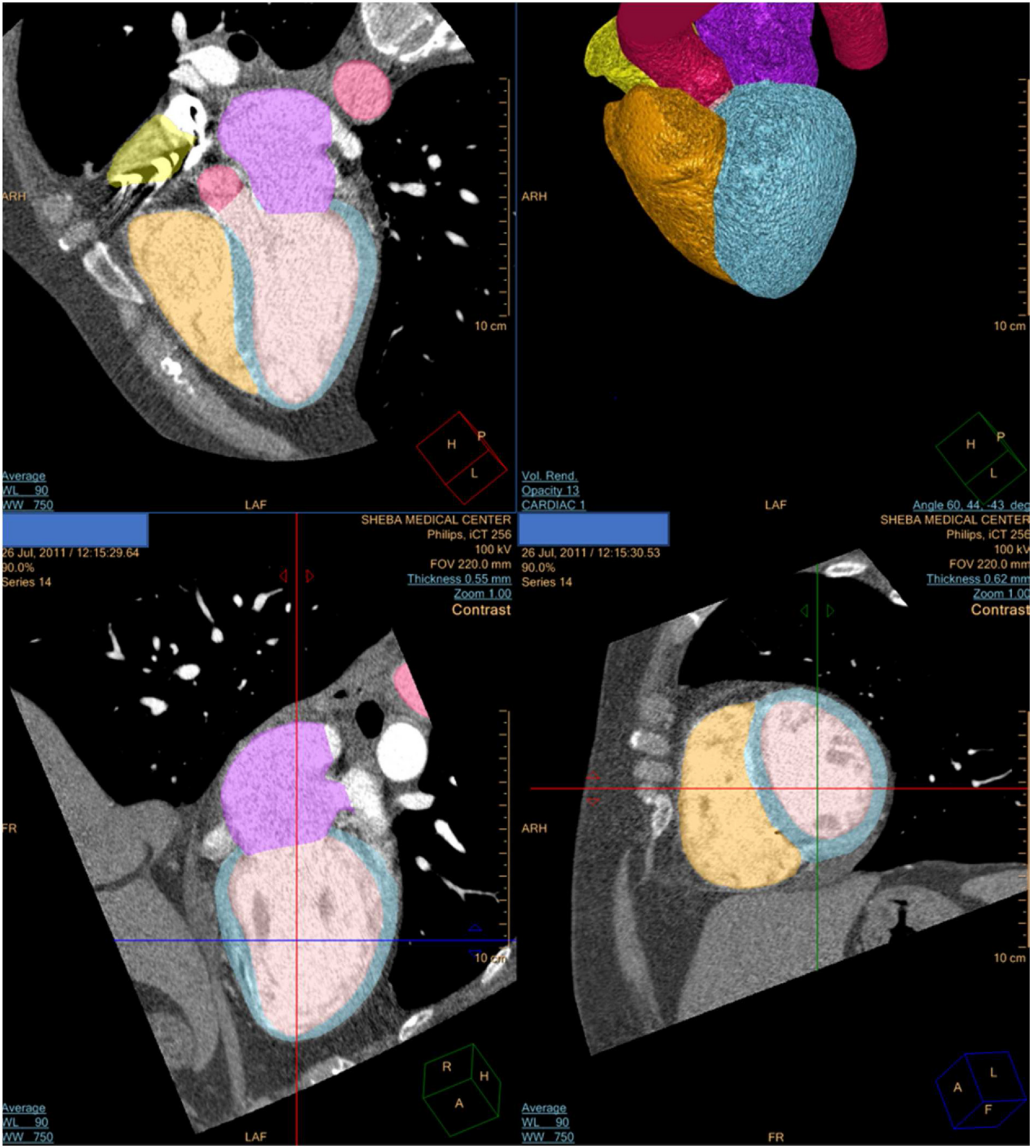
The Cardiac Computed Tomographic Angiography (CCTA) data automatic reconstruction using a dedicated cardiac imaging platform (Comprehensive Cardiac Analysis, Extended Brilliance Workspace (version 4.5); Philips Healthcare).
